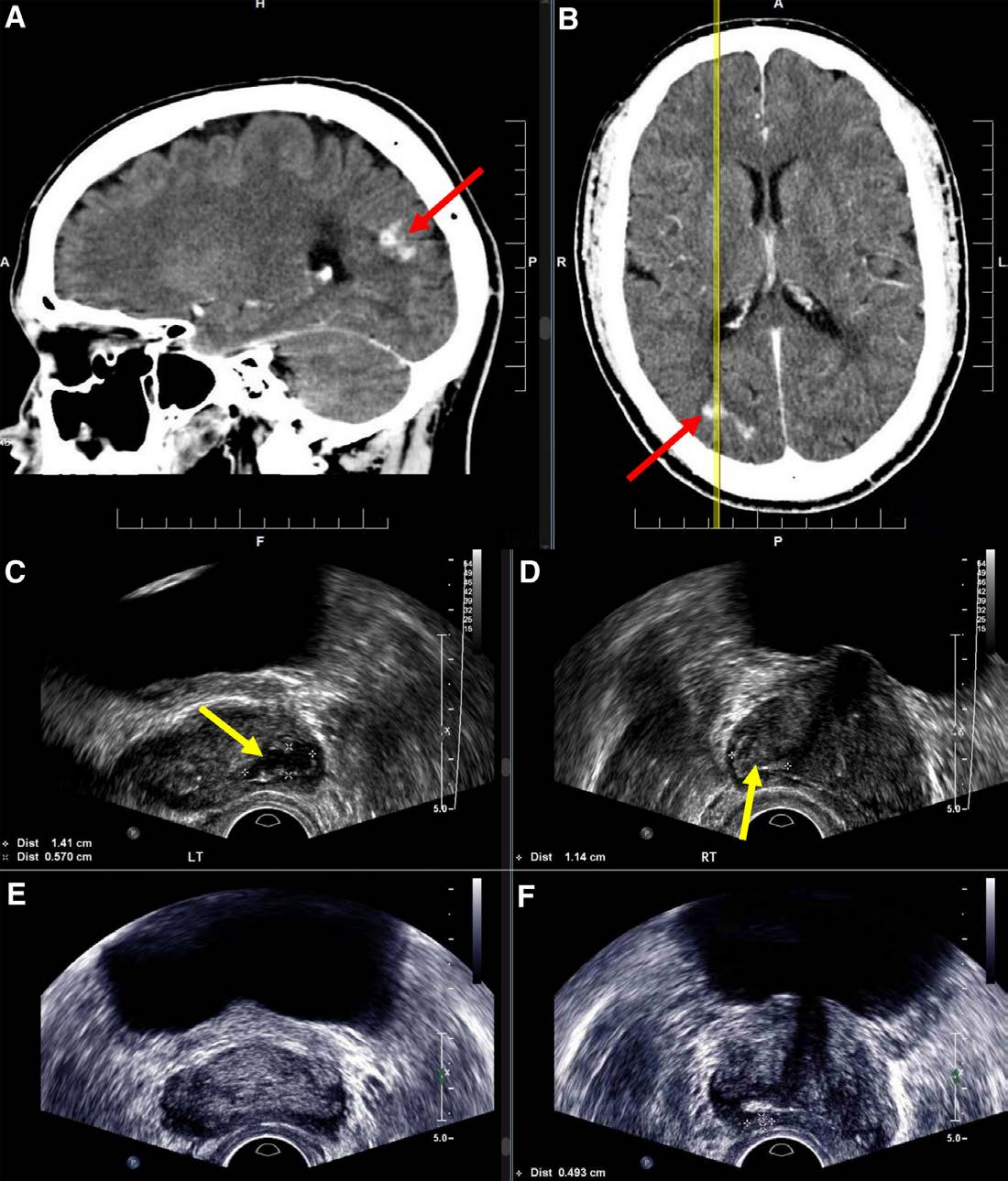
Imaging of case presentation. Contrast-enhanced computed tomography with select sagittal (A) and axial (B) images of the brain (yellow line indicates corresponding sagittal plane), showing enhancing parenchymal lesion (red arrow) with multifocal nodules near the right lateral ventricular horn. Transrectal ultrasound of prostate (C–F). Within 2 wk of diagnosis, left (C) and right (D) prostate bases showing fluid collections (yellow arrow). Two months later, similar imaging views of the prostate base showing resolution of the left-sided (E) and improving right-sided (F) collection.
